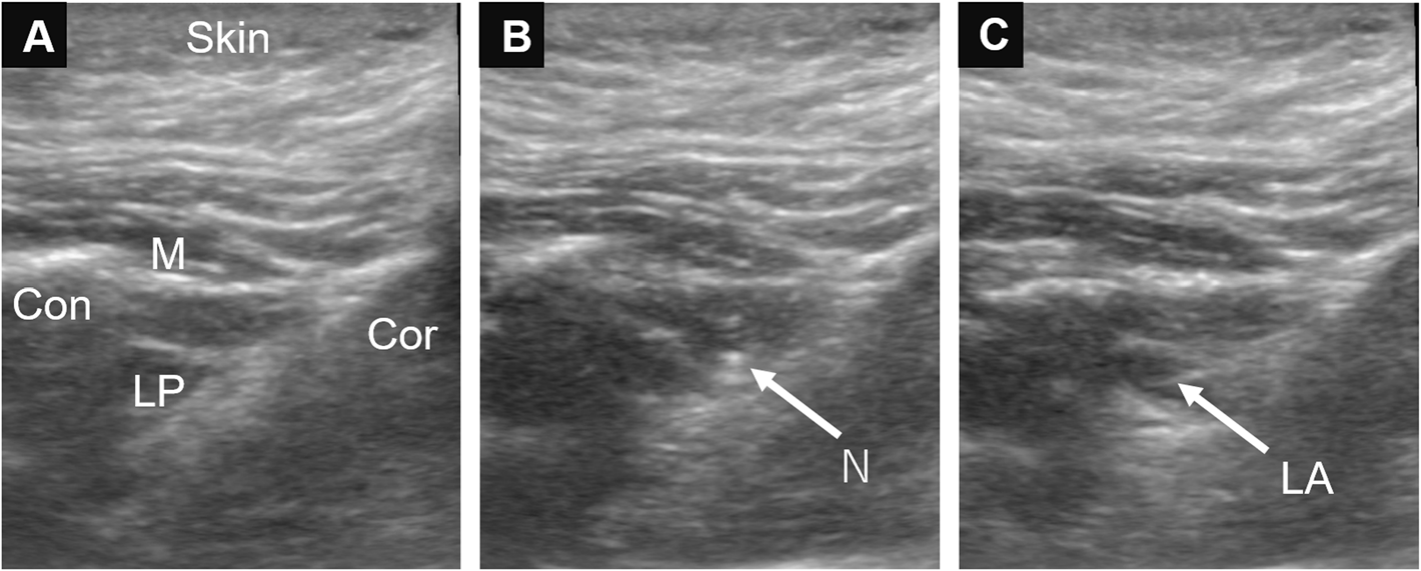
Ultrasound images and technique of inferior alveolar nerve block. A 22-G block needle was inserted using the lateral extraoral technique to infiltrate the inferior alveolar nerve. a, before needle insertion; Cor, coronoid; Con, condyle; M, masseter; LP, lateral pterygoid muscle; b, needle insertion; N, needle; c, after infiltration of local anesthesia; LA, local anesthesia
